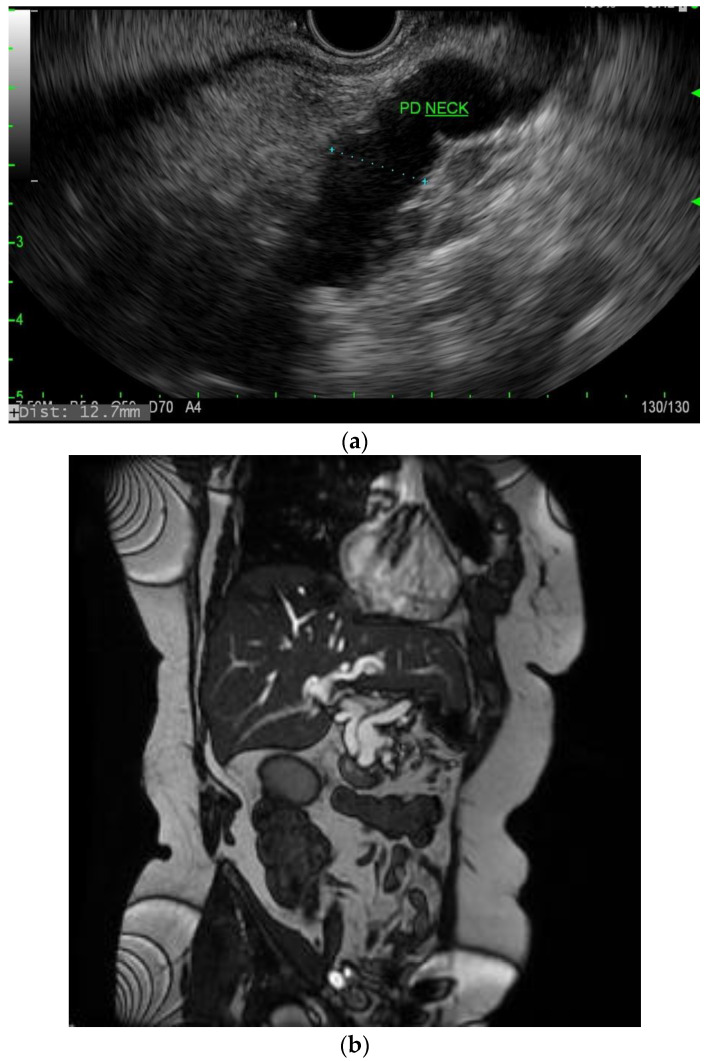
(a) EUS image of main duct IPMN; (b) MRI image of main duct IPMN.
